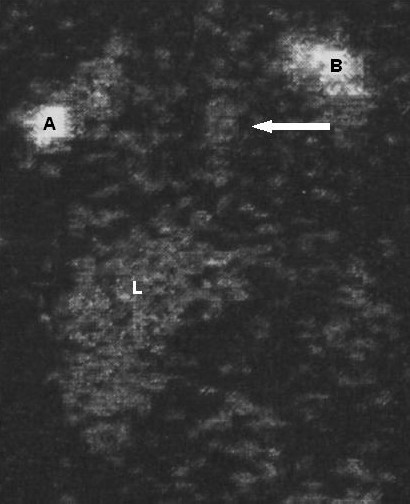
Lymphoscintigraphy. Lymphoscintigraphic study of a patient affected by pulmonary lymphangiectasia and generalized lymphedema showed signs of dermal back-flow in the right lower limb. A and B = patient's hands. L = liver. Arrows point to the thoracic duct.
